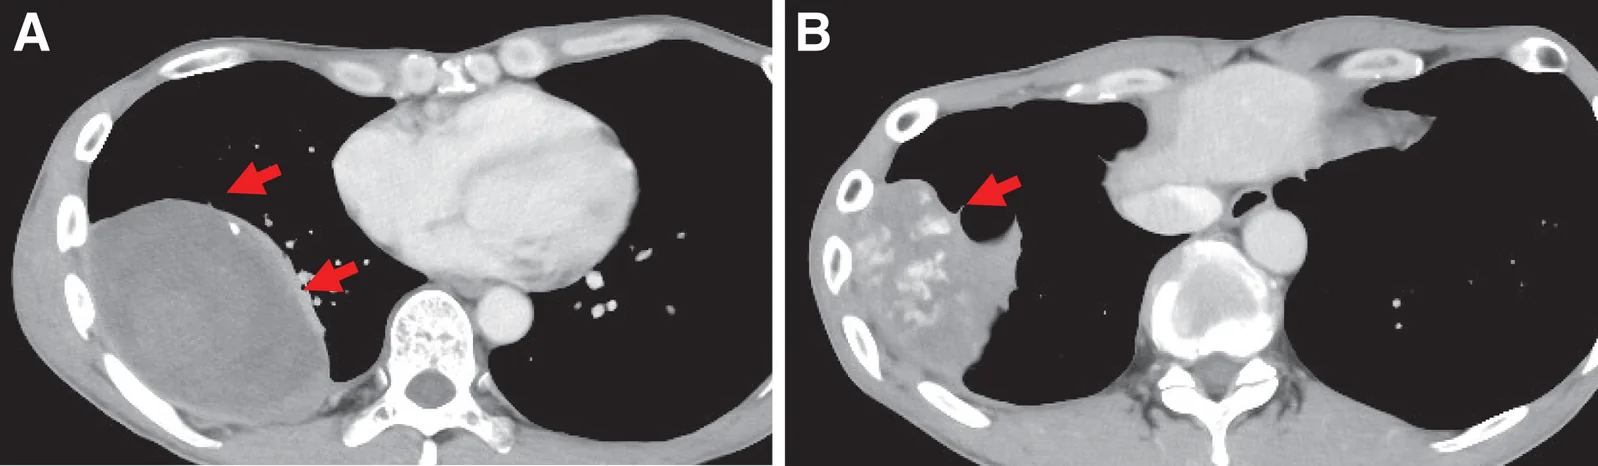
Contrast-enhanced CT findings. (A) The early-phase image showed predominant peripheral enhancement with heterogeneous internal attenuation (arrows). (B) The delayed-phase image showed progressive enhancement of the lesion (arrow).
